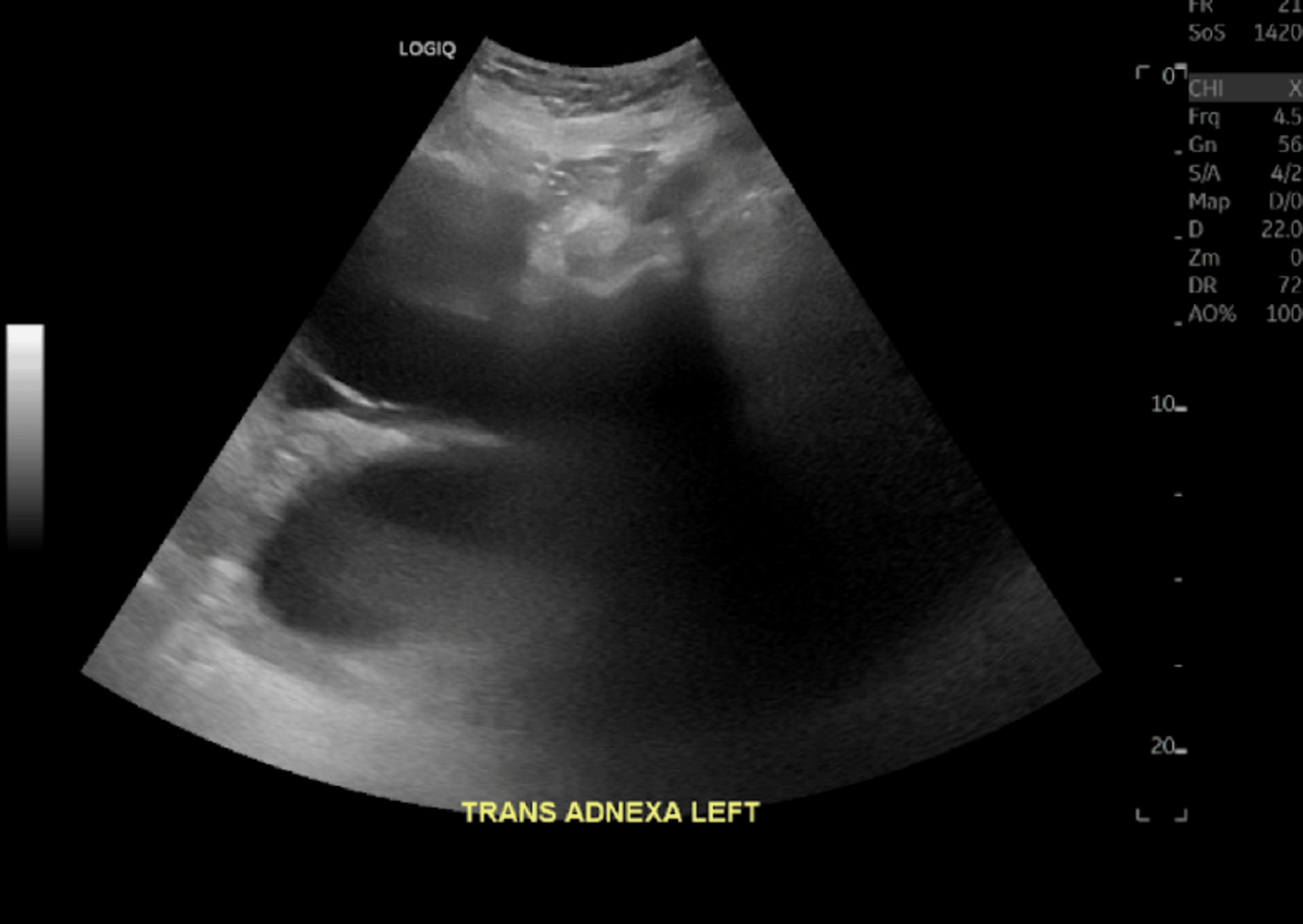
Pelvic ultrasound of the transverse left ovary/adnexa
